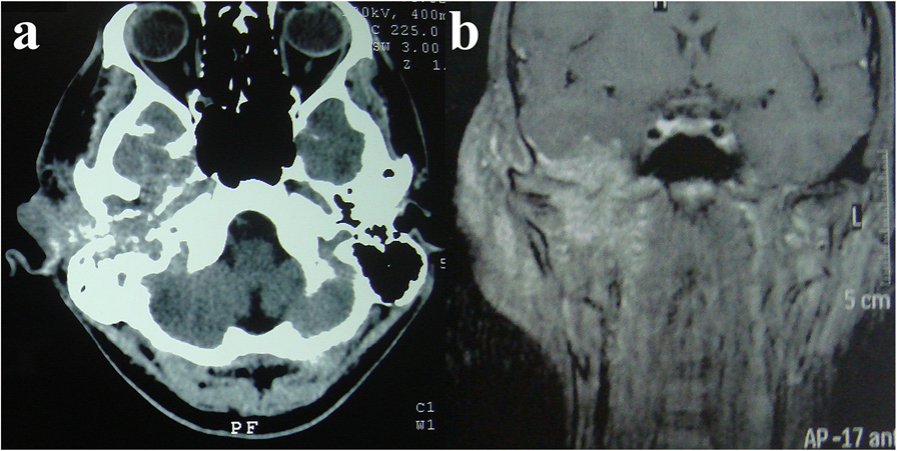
Computed tomography and magnetic resonance imaging. Detailed legend: Computed tomography axial image (a) and magnetic resonance imaging coronal image (b) of the head, performed without (a) and with (b) the administration of contrast material, showing the infiltrative lesion in the external right ear and its relation to anatomical structures.
